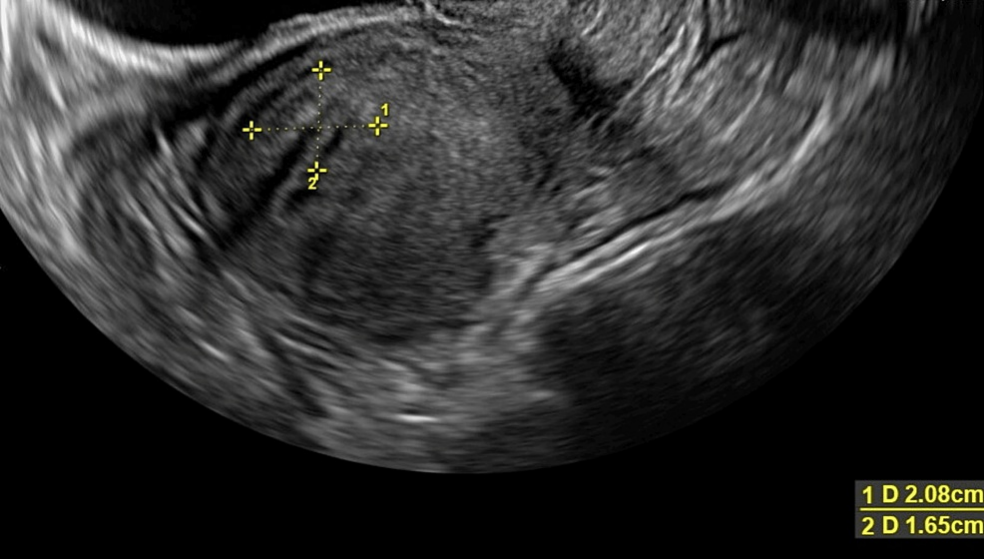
Ultrasound image showing an anterior fibroid measuring 2.1 x 1.7 x 1.9 cm
